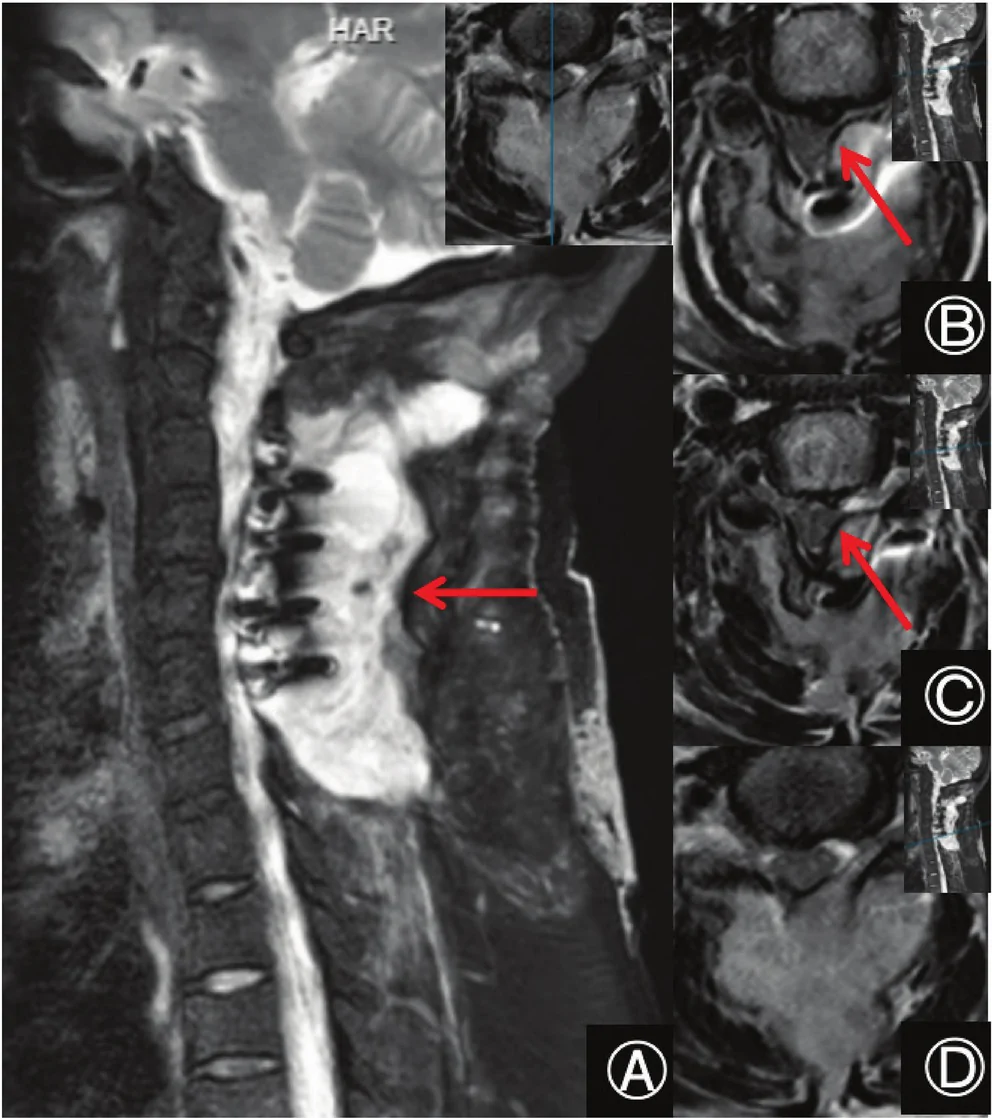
An emergency MRI performed on postoperative Day 7 following hematoma formation revealed a large retrovertebral hematoma, which caused spinal canal stenosis and cord compression at C4–C6 levels.
